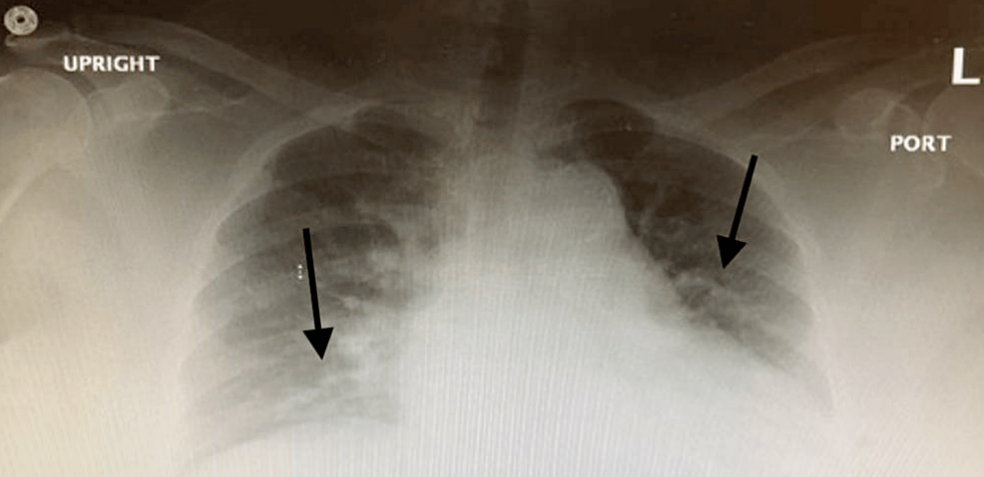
Chest X-ray anteroposterior view showing increased vascular markings
